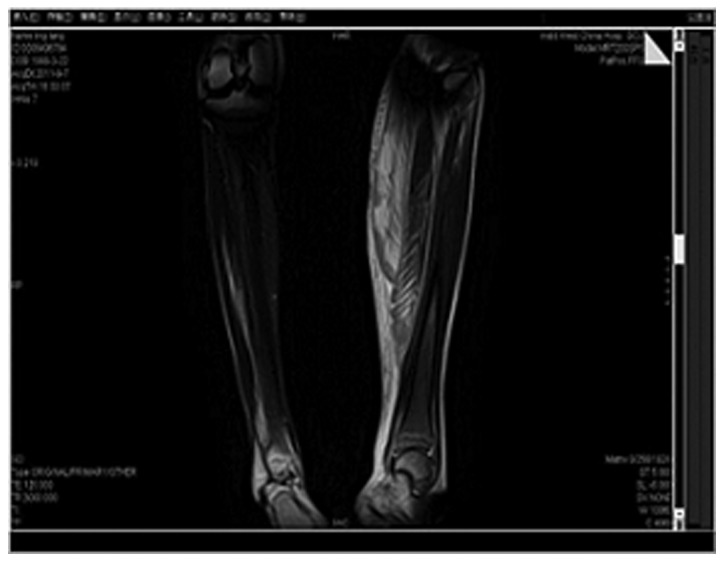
Diffuse infiltrative lesion was hyperintense on T1-weighted image and showed enhancement.
